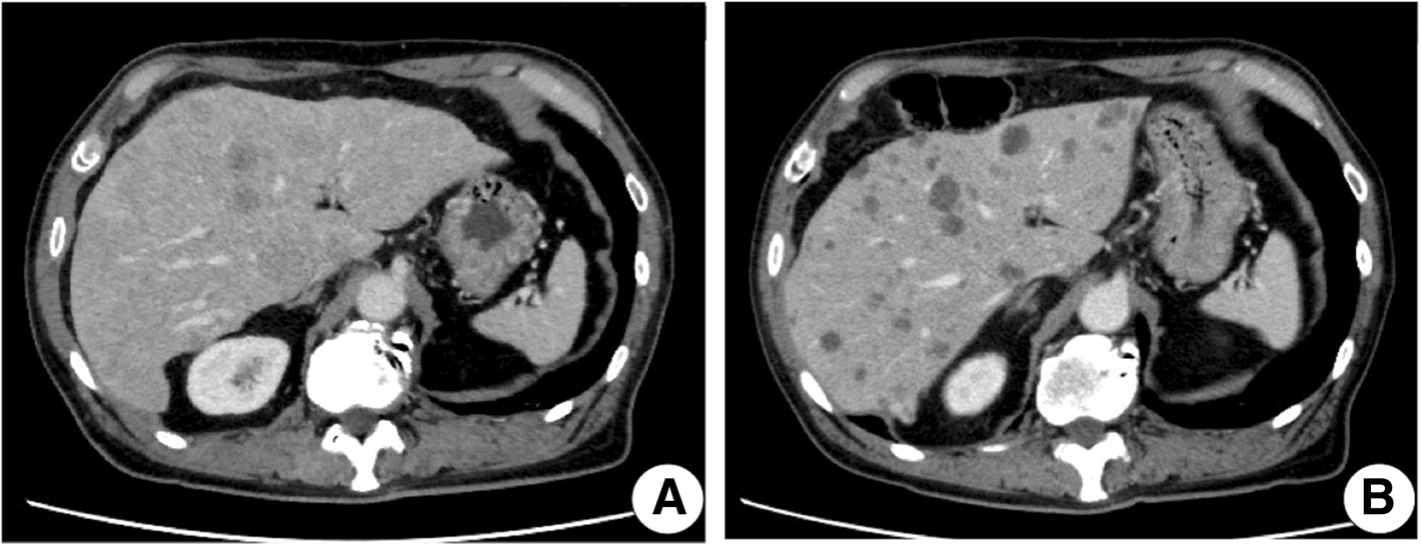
CT scan showed diffuse liver metastases before treatment (a); cystic morphologically changed after 4 courses of mFOLFOX6 (b)
